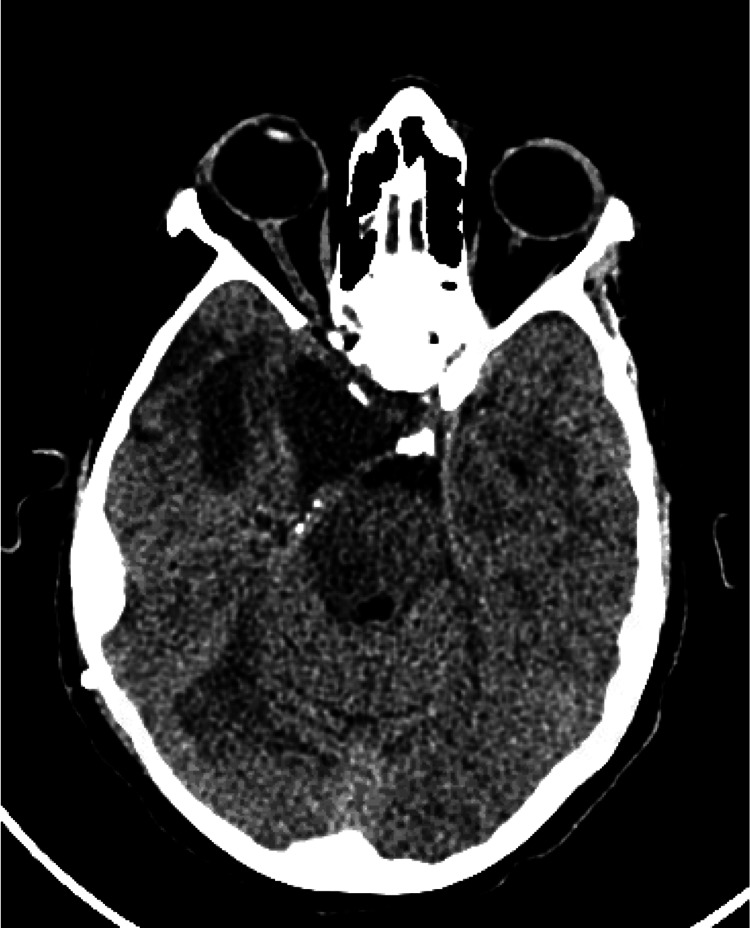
Plain CT brain post-repair, demonstrating resolution of pneumocephalus following endoscopic repair
